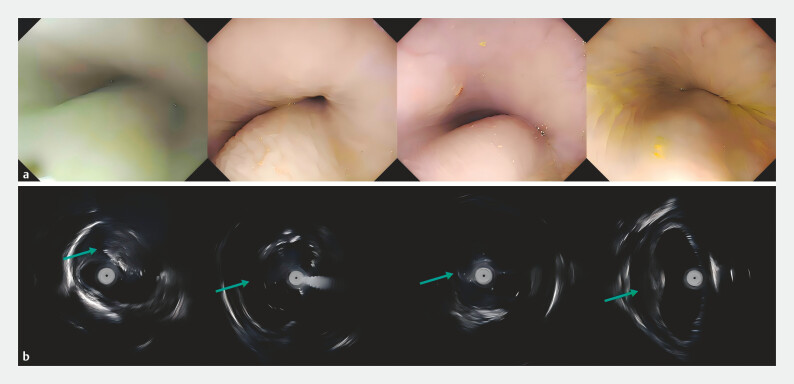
Endoscopic and endosonographic follow-ups.
